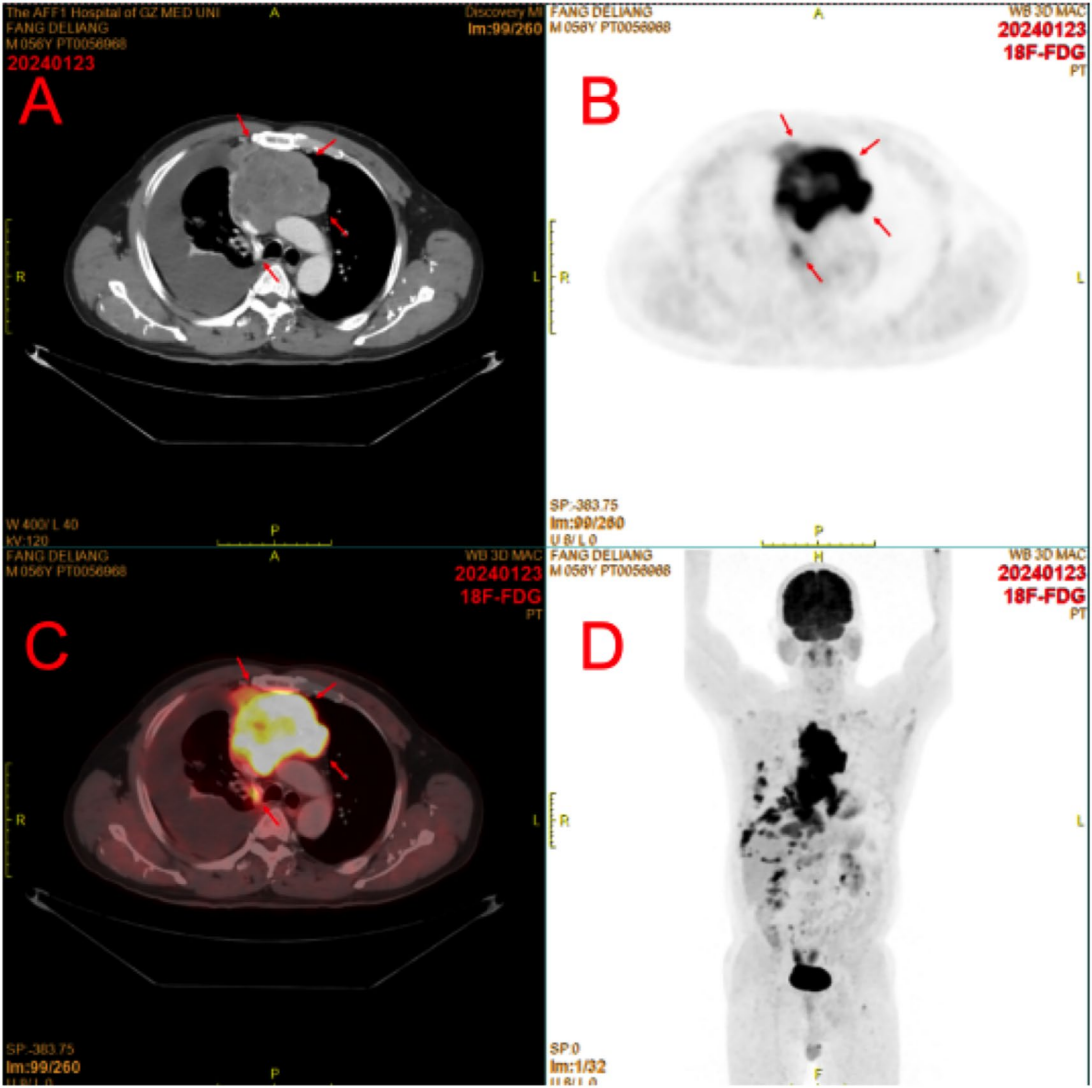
PET‐CT imaging results of the patient with primary malignant melanoma of the mediastinum. (A–C) High metabolic tumor lesions in the anterior mediastinum, with CT enhancement scan showing mild heterogeneous enhancement. Extensive tumor metastasis of the right pleura, accompanied by a large amount of pleural effusion on the right side. (D) Multiple tumor metastases in the right pulmonary hilum, hepatogastric space, around the abdominal aorta, and around the mesentery.
